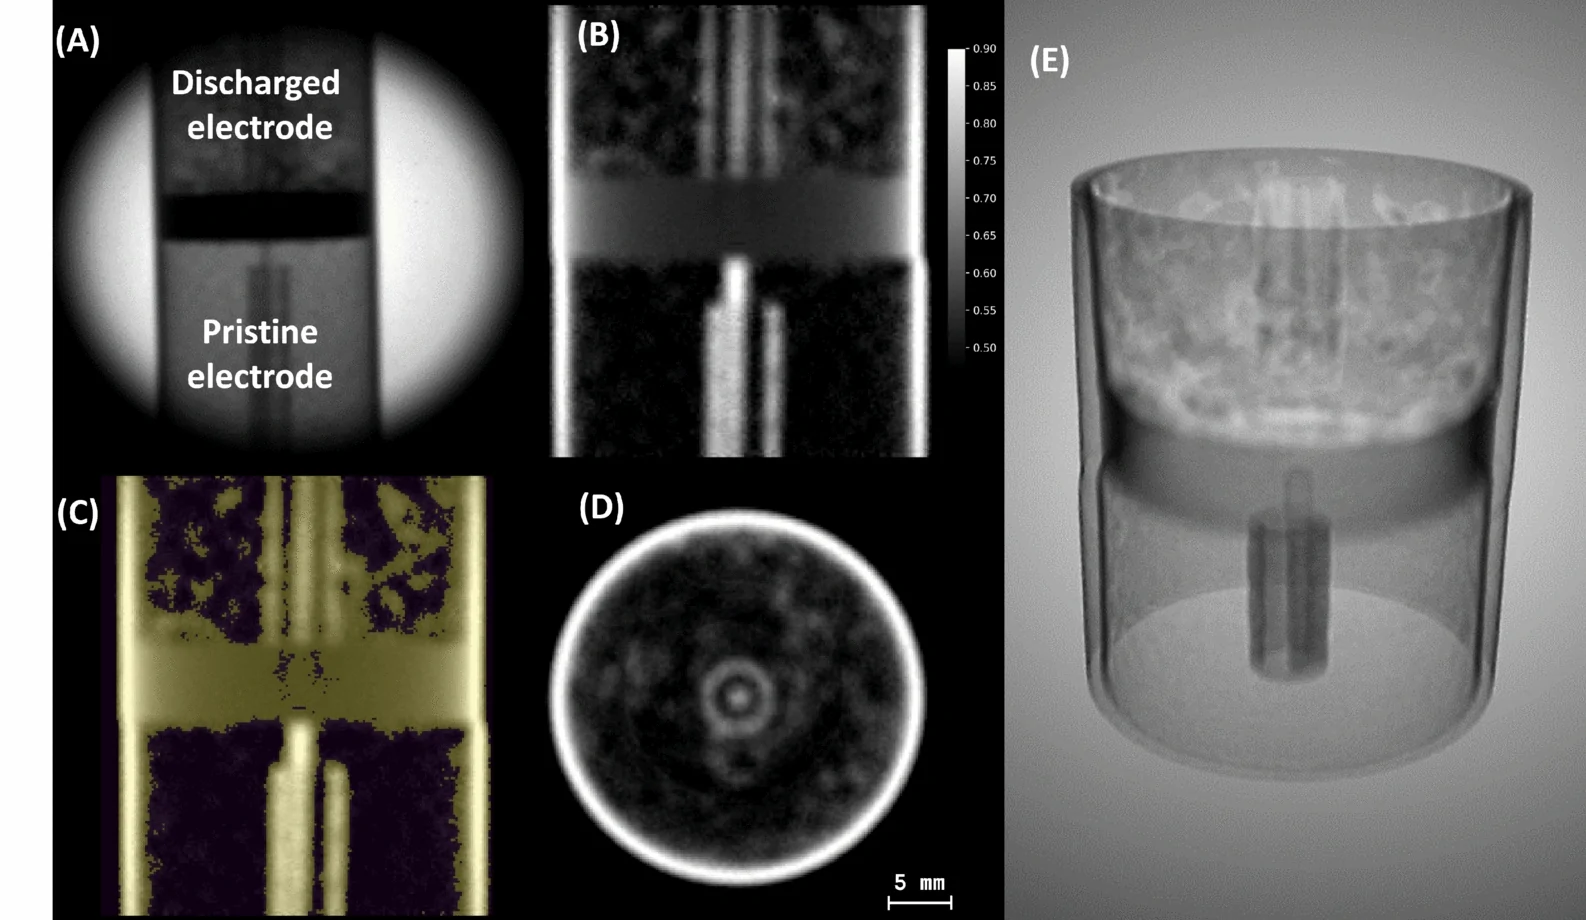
(A) Representative neutron radiography of the pristine and discharged electrodes. (B–D) Sagittal and axial slices of the 3D tomographic reconstruction. The light grey regions correspond to hydrogenated discharge products. The red segmentation in (C) indicates the most attenuating materials. (E) Volume rendering, highlighting hydrogenated species.
